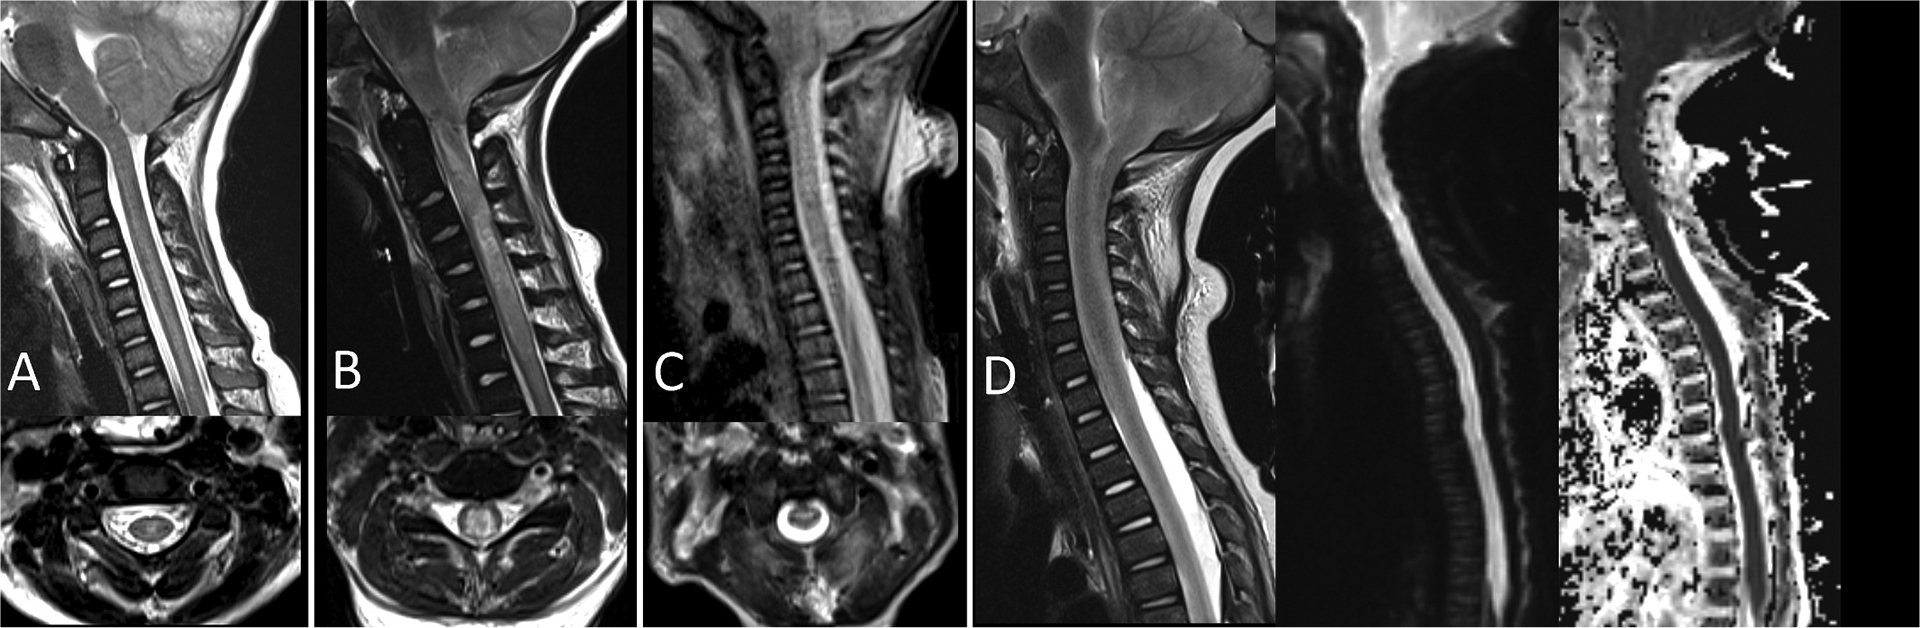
Various degrees of spinal cord ischemic injury in three patients after cardiac arrest. A. A 5-year-old patient after cardiac arrest. Sagittal and axial T2-weighted images demonstrate faint hyperintense signal in the central cervical spinal cord gray matter. B. A 7-year-old patient after cardiac arrest. Sagittal and axial T2-weighted images demonstrate tonsillar herniation, marked cervical spinal cord swelling, and marked hyperintense signal in the central spinal cord involving both gray and white matter. C. A 5-month-old patient after cardiac arrest of unknown etiology but suspected to be sudden infant death syndrome. Sagittal and axial T2-weighted images demonstrate HISCI at the level of C1-C3 without evidence of trauma. D. A 10-month-old patient after cardiac arrest and suspicion of non-accidental trauma. Sagittal T2-weighted, sagittal diffusion-weighted, and sagittal ADC (apparent diffusion coefficient) images demonstrate marked tonsillar herniation, with diffuse cord swelling and ischemic injury of the entirety of the spinal cord.
